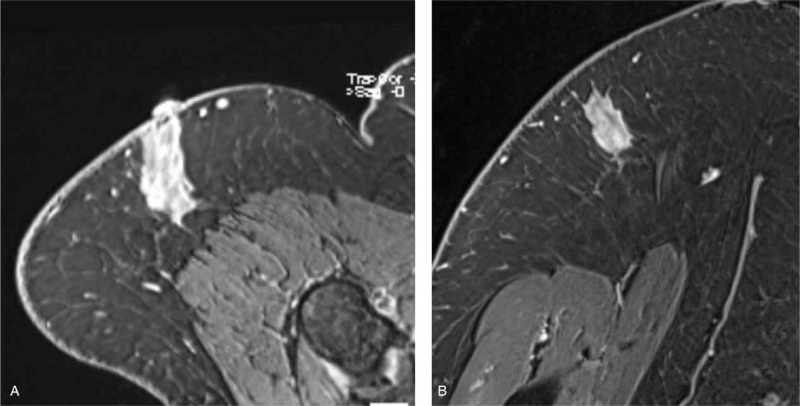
Follow-up MRI, 1 month following initial MRI. A) Axial VIBE sequence with gadolinium contrast; B) coronal VIBE sequence with gadolinium contrast.
